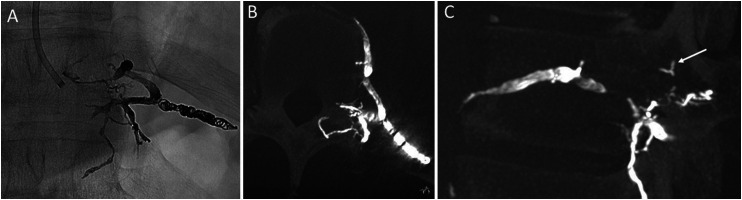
Post-embolization single-shot DSA acquisition (A) and cone-beam computed tomography (CT) images (B, C), demonstrating hyperdense embolic material in the draining T10–T11 intersegmental vein and in multiple periforaminal veins, including those visualized on the left lateral decubitus CT myelography (C, arrow).
